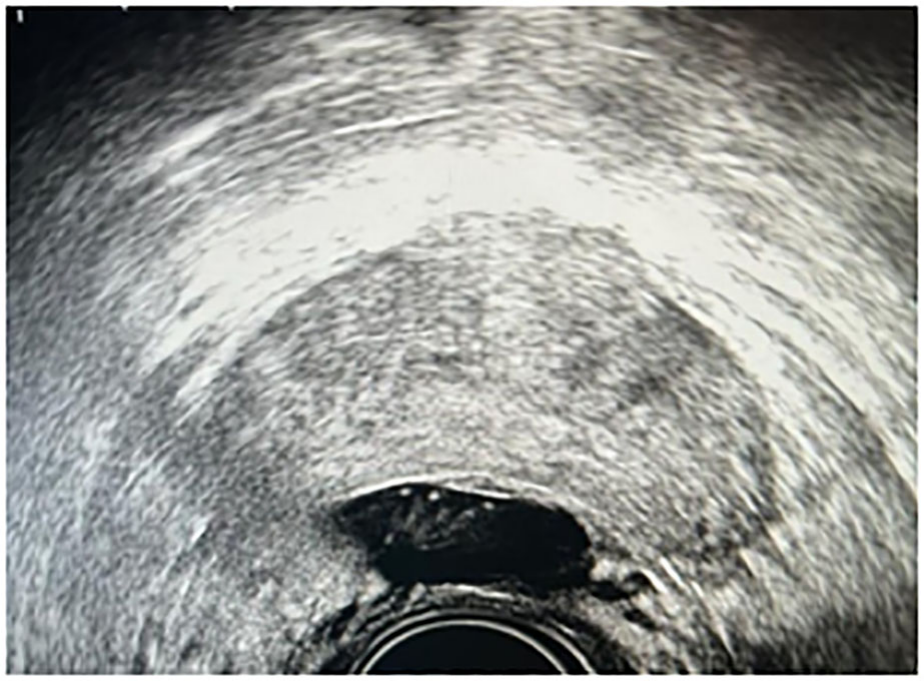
Ultrasound image showing the right placement of hydrogel between the prostate and the rectal wall.
